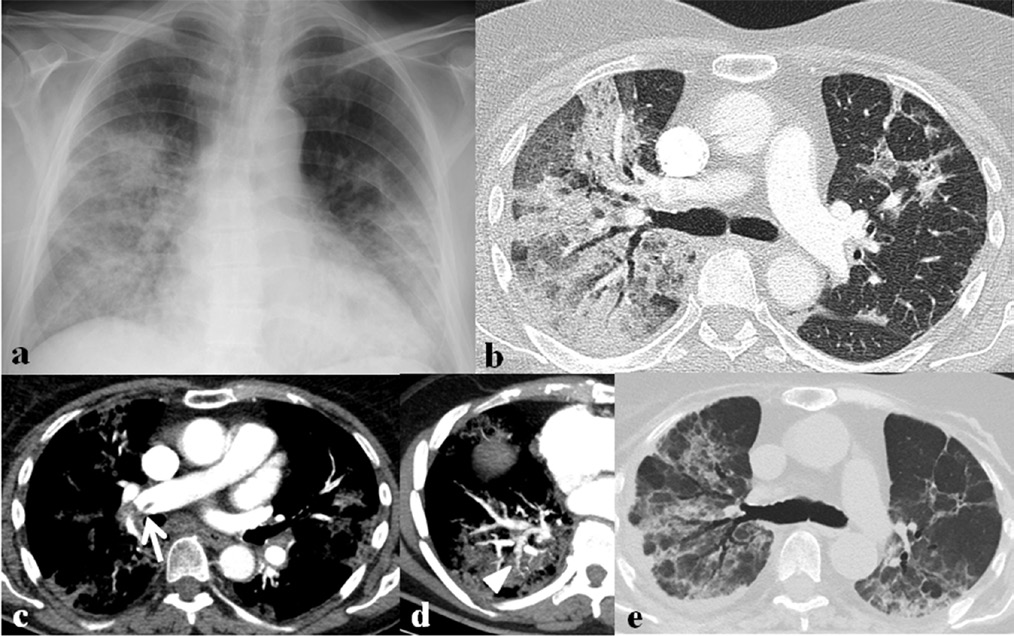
A 78-year-old female patient with SARS-CoV-2 infection. (a) Initial chest radiograph shows bilateral diffuse consolidation in both lungs. (b) Lung window image of contrast-enhanced chest CT scan obtained on the same day with (a) shows mixed ground-glass opacities (GGOs) and consolidation in both lungs. (c, d) Mediastinal window images of contrast-enhanced chest CT scan obtained on the same day with (a) show thromboembolism (arrow) involving right interlobar pulmonary artery and irregular-walled vessels (arrowhead) toward consolidation in right lower lobe. (e) Follow-up chest CT scan after 1 month of (a) shows decreased extent of mixed GGOs and consolidation with remnant multifocal patchy GGOs, parenchymal bands, traction bronchiectasis, and irregular interface, suggesting pulmonary fibrosis.
